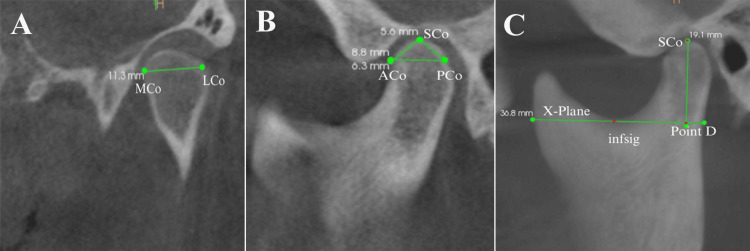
Condylar measurements in mm: (A) Width - measured in the coronal plane as the linear distance between the most medial mandibular condylar point (MCo) and the most lateral mandibular condylar point (LCo). (B) Length - measured in the sagittal plane as the distance between the anterior (ACo) and posterior (PCo) mandibular condylar points, which were identified as 4 mm inferior to the superior condylar point (SCo). (C) Height - measured as the vertical distance from the intersection of a horizontal tangent drawn through the most inferior point of the sigmoid notch (infsig) to the posterior border of the ramus, up to the superior-most point of the condyle (SCo). This figure depicts cone-beam computed tomographic images of a patient included in this study and used with the patients' permission.
